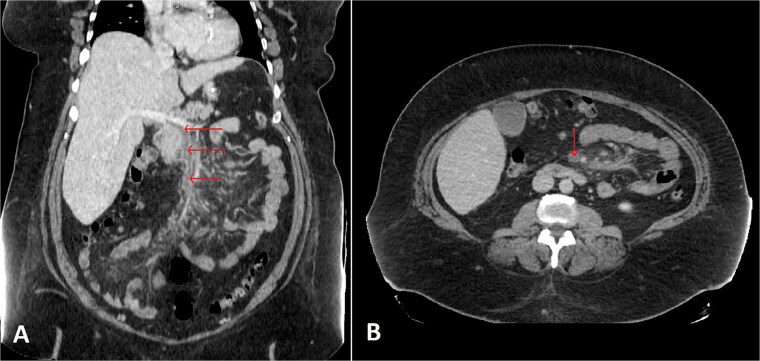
Selected coronal (A) and axial (B) cuts of the patient’s abdomen CT scan with IV contrast showing intra-luminal filling defect in the SMV, extending proximally to the proximal part of the main portal vein (arrows) associated with surrounding mesenteric fat haziness, representing portomesenteric venous thrombosis.
